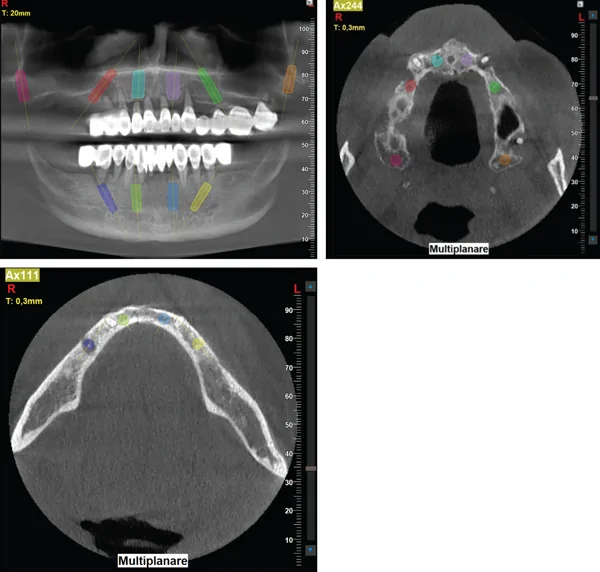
Rehabilitation project on the CBCT DICOM viewer (NewTom NNT).
